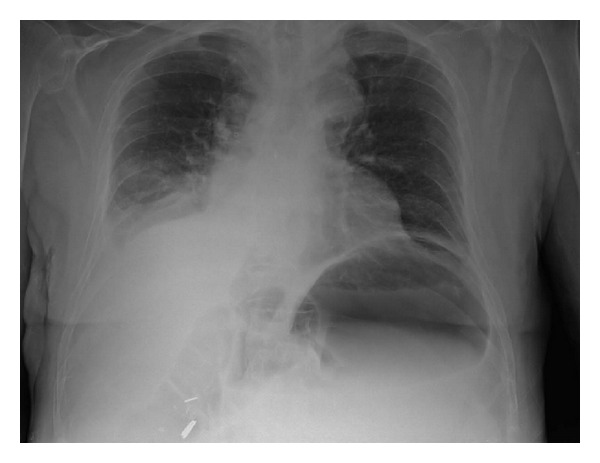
X-ray chest and abdomen showing opacification in the left hypochondrium.
